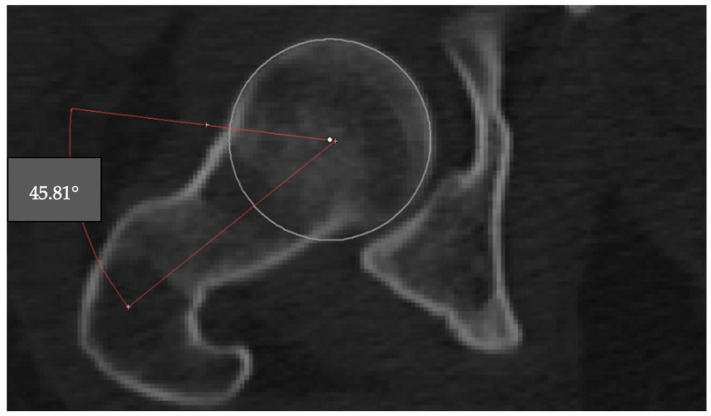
Measurement of the alpha angle on an axial CT slice. The angle is formed between the femoral neck axis and a line drawn from the femoral head center to the point where the head contour deviates from the best-fit circle (45.81°).
